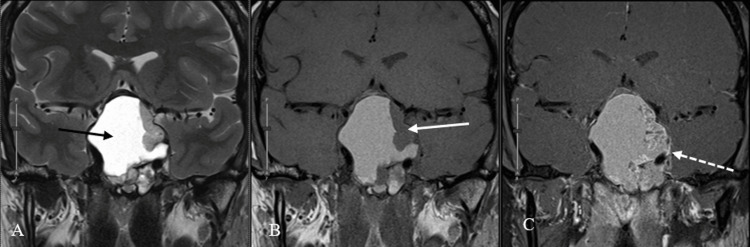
Coronal magnetic resonance images of varying sequences. Coronal T2 (A), T1 (B), and T1 post-contrast (C) magnetic resonance images. This lesion is composed of cystic (black arrow) and solid (white arrow) components evidenced by non-enhancing central T2 hyperintensity and enhancement within the peripheral nodular components (dotted white arrow).
